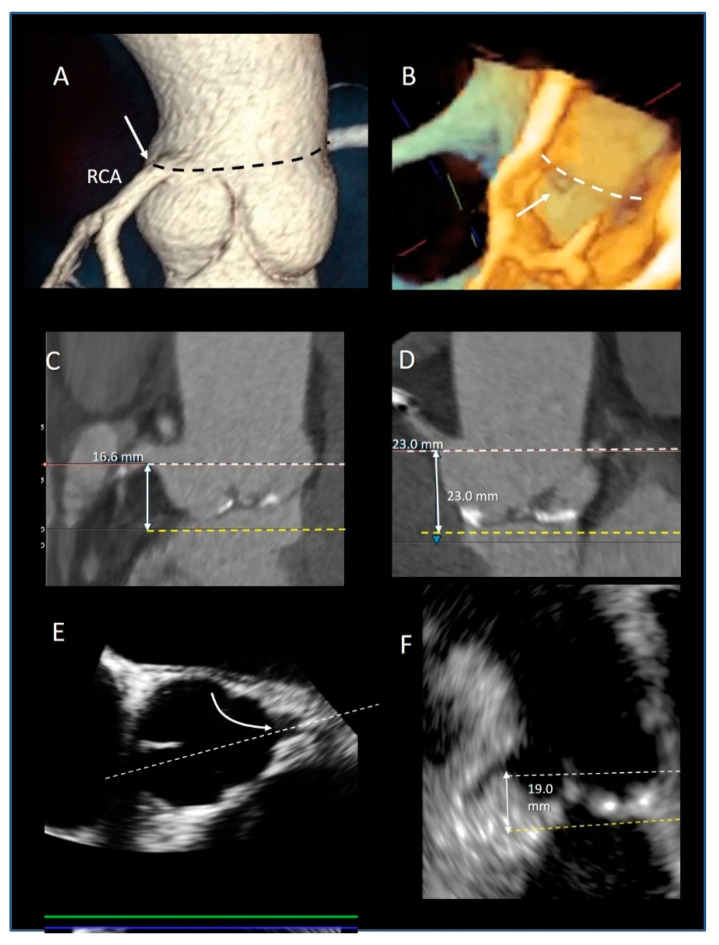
(A) CT Volume rendering modality showing the origin of right coronary artery arise at the level of sinutubular junction (black dotted line). (B) The 3D TEE longitudinal cross-section showing the orifice of coronary artery (arrow) at level of sinutubular junction (white dotted line). (C,D) CT multiplanar image modality showing the measurements of the distance (double-headed arrow) between the coronary ostia (white dotted line) and the virtual annulus (yellow dotted line). (E) The 2D TEE multiplanar reconstruction derived by 3D data set in short-axis view of the aorta showing the orifice of LAD (curved arrow). The white dotted line indicates the longitudinal cross-section plane that shows the image of the panel. (F) From this image, the distance between the coronary ostia (white dotted line) and the virtual annulus (yellow dotted line) can be measured (double-headed arrows).
